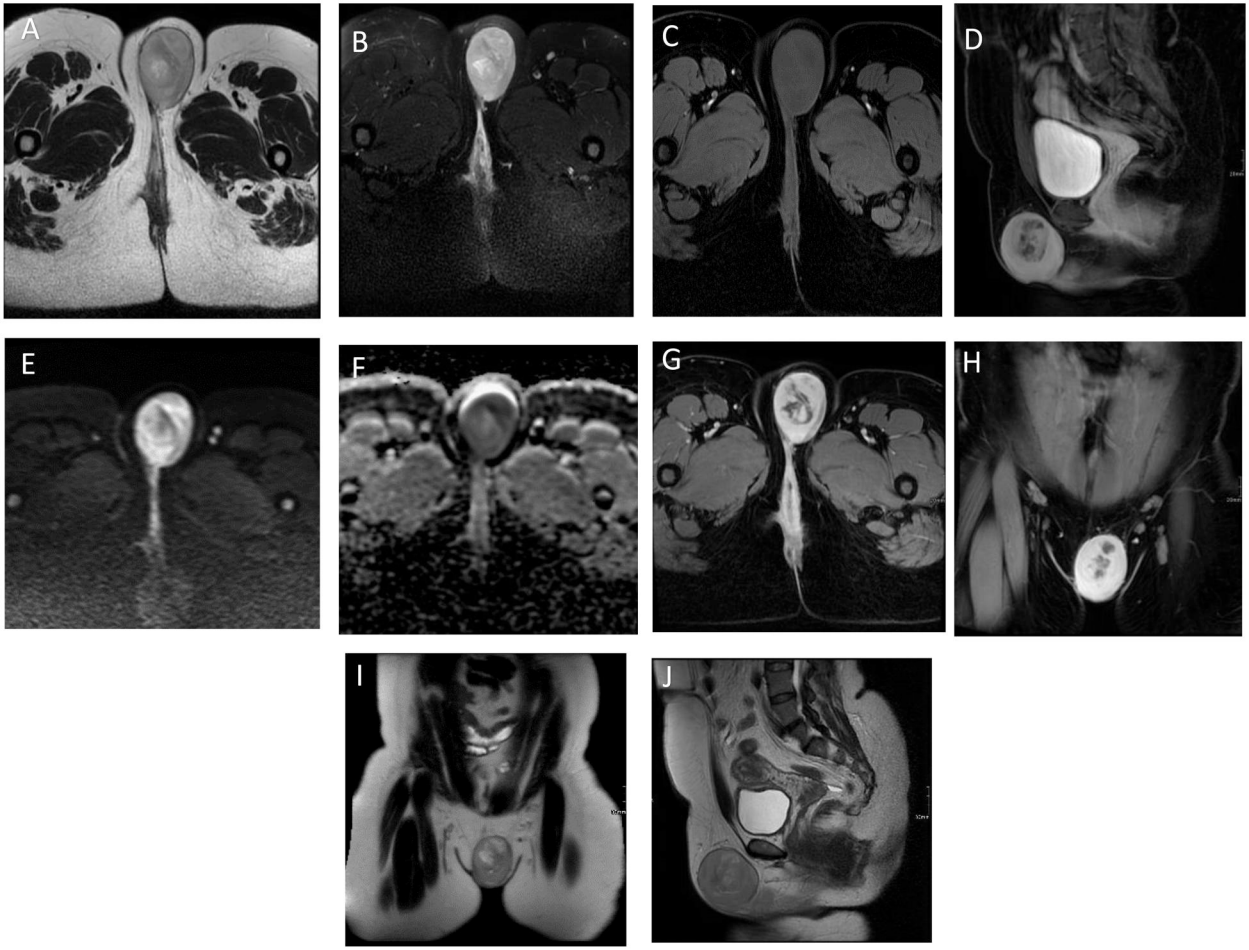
Multiple selected images from different sequences of the contrast-enhanced MRI of the pelvis, displayed in axial, coronal, and sagittal planes, showing: left-sided subcutaneous lobulated vulvar mass measuring 5.8 × 6.8 cm that extends to the labia majora and more inferiorly towards the left gluteal fold (image I), with close proximity to the distal urethra and clitoris without obvious invasion. The mass exhibits a smooth contour, intermediate signal intensity (SI) on T2-weighted images (images A, I, J) with no fat suppression on T2/STIR (image B), low SI on T1-fat saturated images (image C), diffusion restriction (image E, F), and intense progressive enhancement in the post-contrast sequences (images B, D, H).
